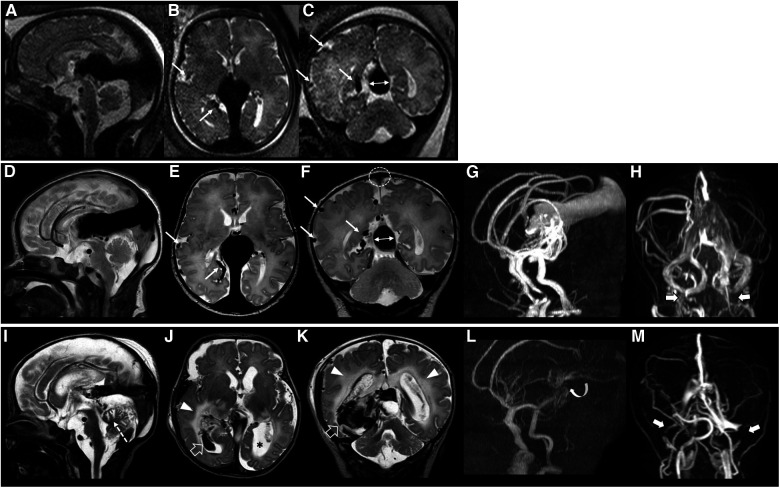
Fetal brain MRI performed at 37 gestational weeks (A–C), post-natal brain MRI performed at birth (D–H), and last brain MRI performed at 5 days of age (I–M), 3 days after endovascular treatment in patient #40 with a dismal prognosis. Fetal and post-natal sagittal (A,D), axial (B,E) and coronal (C,F) T2-weighted images demonstrate a VGAM associated with pseudofeeders from the right middle cerebral artery (thin arrows) and a high SS-MD (thin arrows with double heads, 13.5 mm). Note on post-natal MRI that there is also stenosis of the superior sagittal sinus (dotted circle) with SSS index of 2.2. MR arterial (G) and venous (H) angiography reveal a large VGAM pouch with feeders from the choroidal and anterior cerebral arteries, with stenosis of the jugular bulbs, more pronounced on the left (thick arrows). Last brain MRI (I–K) and MR arterial and venous angiography (L,M) show marked reduction of the VGAM pouch and venous efferents after embolization (curved arrow). However, there is a major complication characterized by intracranial bleeding (empty arrows), white matter edema (arrowheads) and cerebellar vermis ischemic lesion (dotted arrow). Note the ventricular dilatation, especially on the left (asterisk), and the occlusion of the transverse-sigmoid sinuses (thick arrows). The patient died soon after this last MRI.
